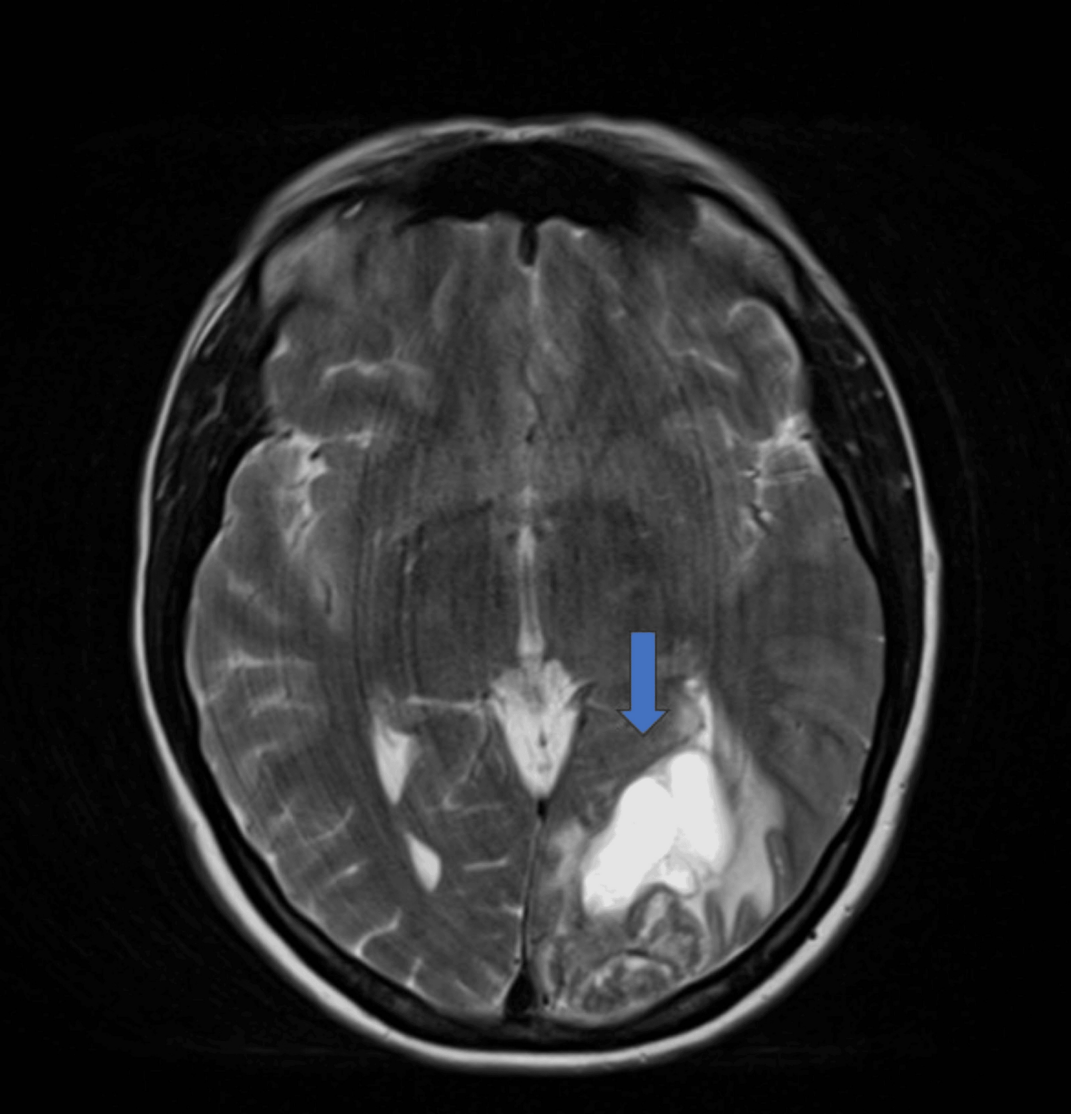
Magnetic resonance imaging (MRI) showing a solitary/cystic lesion in the left parieto-occipital lobe with peripheral enhancement and hemorrhage within
